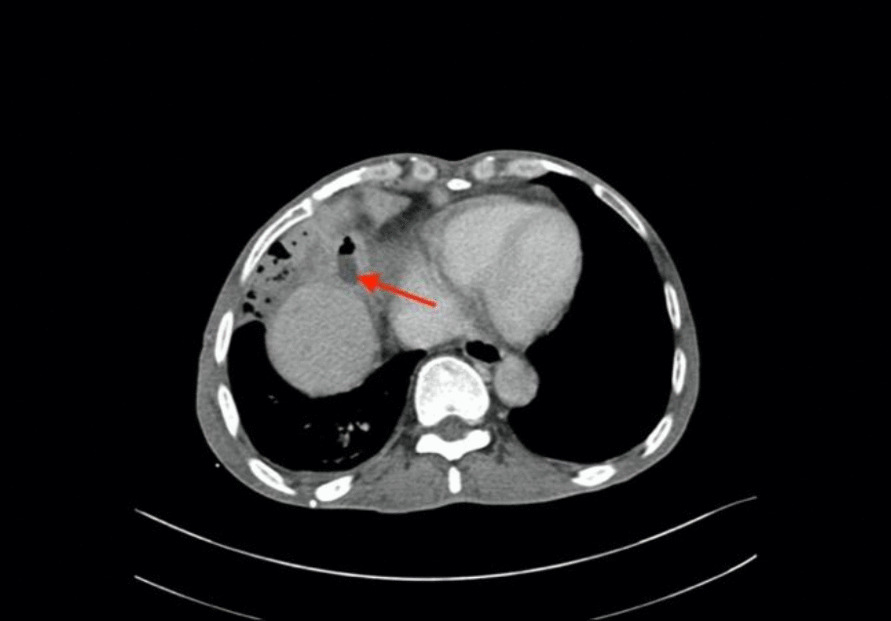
The BBF seen on the CT scan of the abdomen (red arrow)
